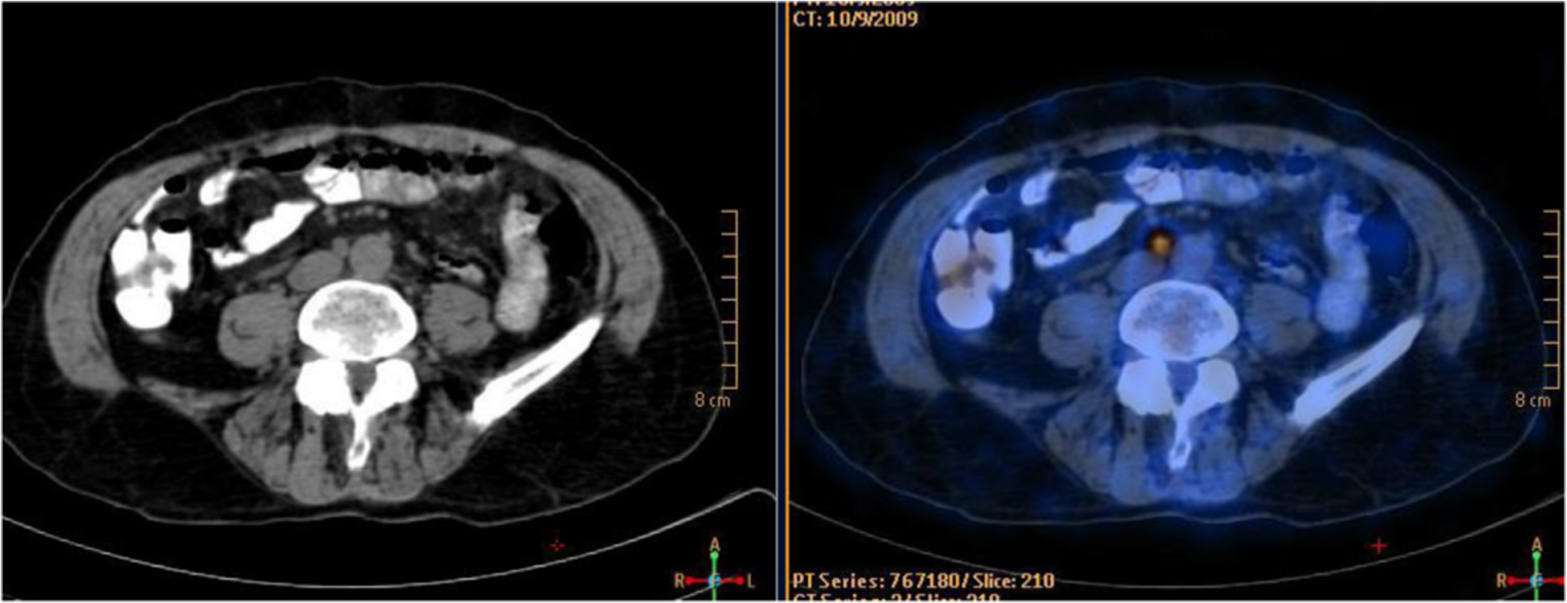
Transaxial CT and fused PET/CT images of the same patient.^18^F-FDG PET/CT showed intense ^18^F-FDG uptake (SUVmax: 5.2) in millimeter-sized aortocaval lymph nodes which were not reported as pathological on ceCT.
